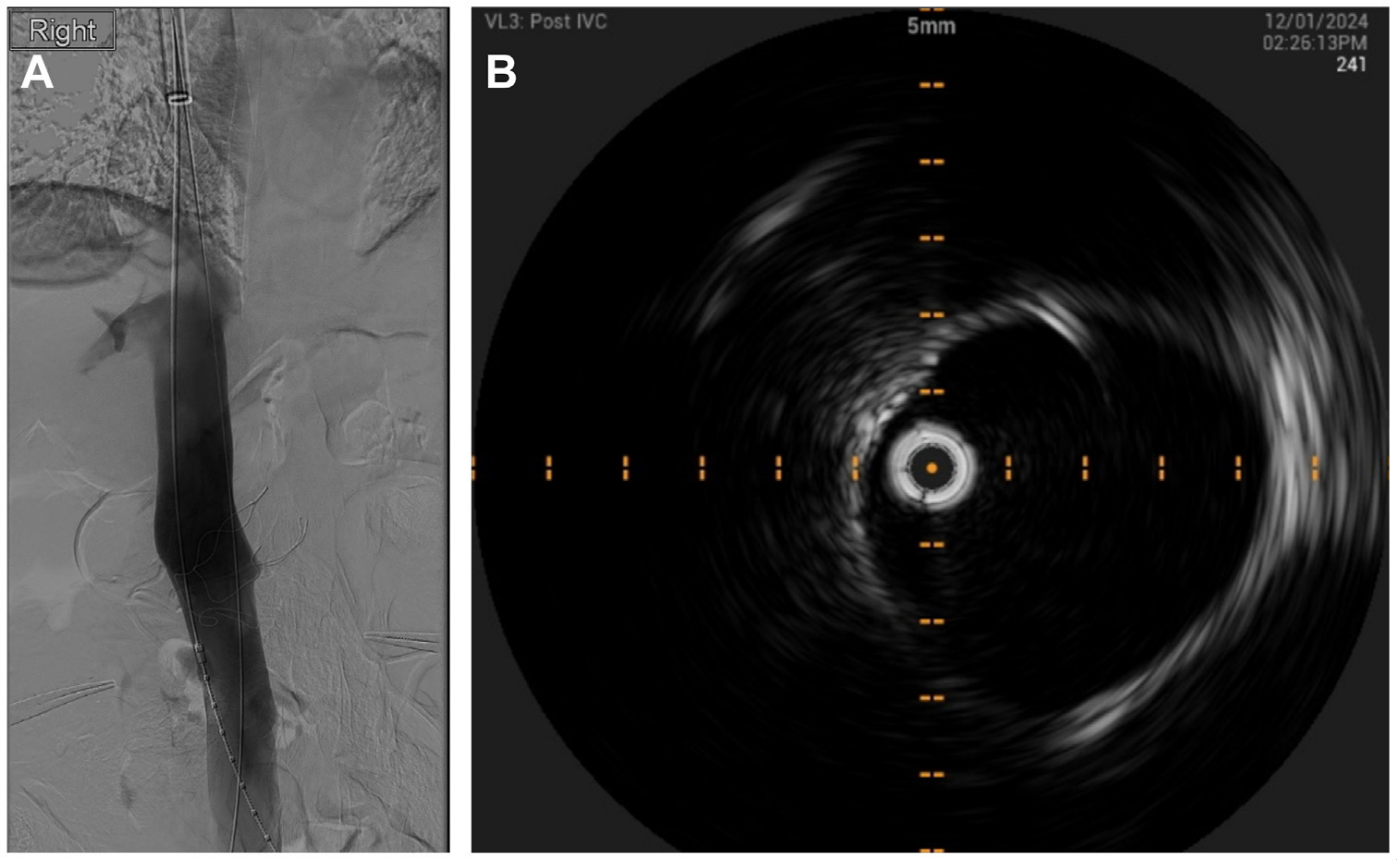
A, Digital subtraction venography demonstrating complete clearance of the pararenal inferior vena cava (*IVC*) mass following endovascular mechanical thrombectomy. B, Intravenous ultrasound (*IVUS*) of the pararenal inferior vena cava (*IVC*) demonstrating a widely patent caval lumen following endovascular mechanical thrombectomy.
